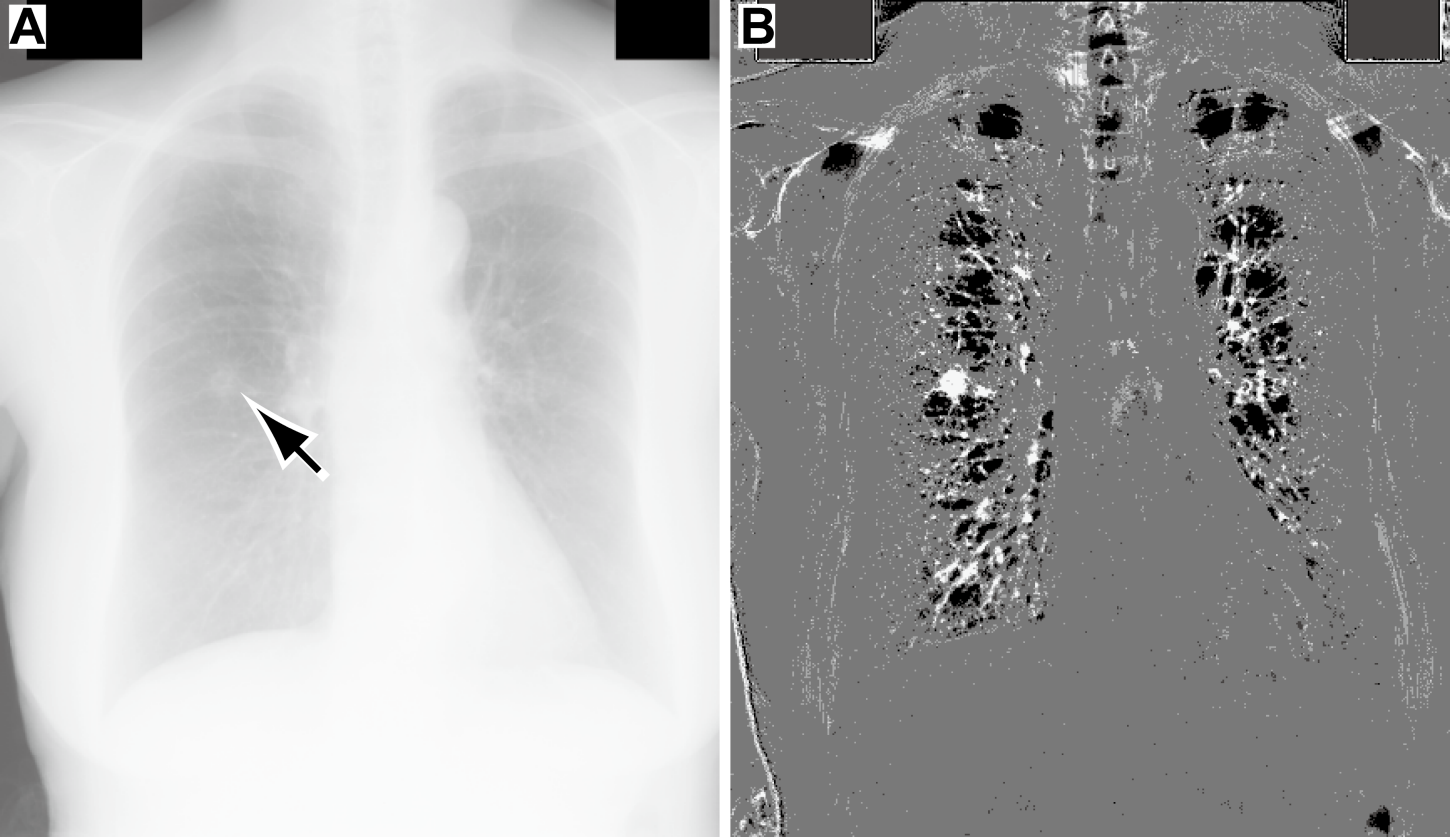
Image enhancement achieved by the proposed method for a nodule in a chest radiographic image. (A) Original chest radiographic image (*JPCLN044*); the location of the single lesion (pulmonary nodule) is indicated by the arrow. (B) Enhanced image obtained by the proposed method.
